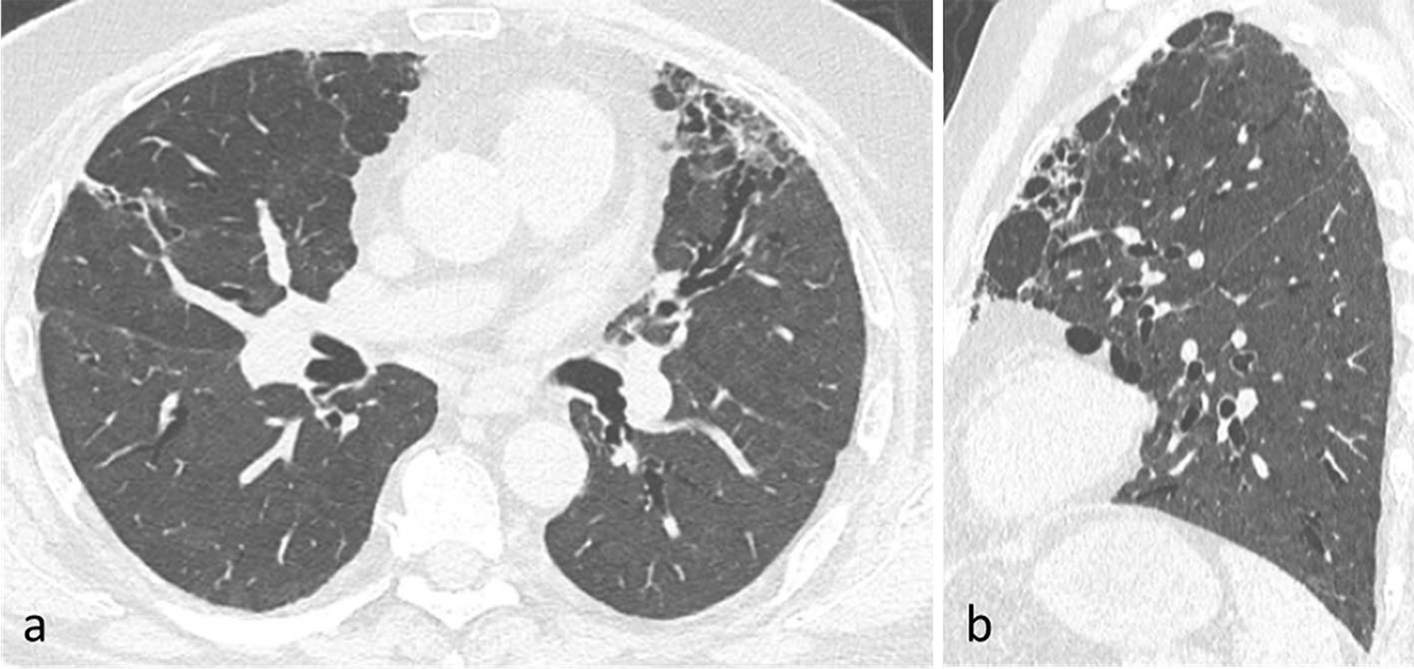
axial (a) and sagittal (b) CT images representing changes typical of post-ventilatory damage, characterized by cicatricial emphysema, parenchymal bands, and traction bronchiectasis in the anterior portions of upper lobes.
